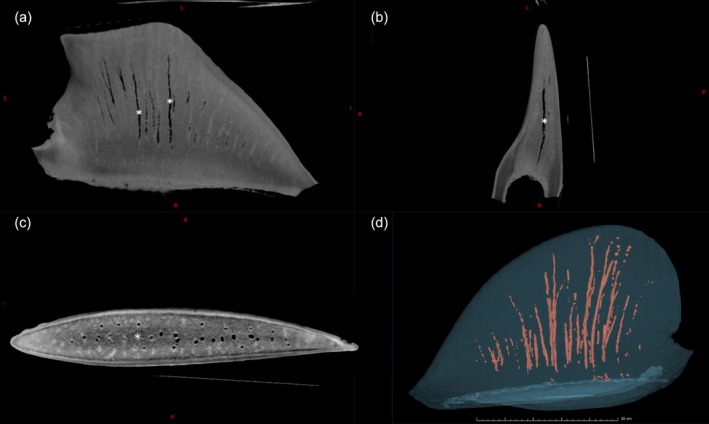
CT scan of the dorsal fin of spectacled porpoise (*P. dioptrica*) KS14‐45Pd/X2020.76. (a), (b) and (c) show the sagittal, transversal, and axial planes at its base, respectively. (d) The 3D reconstruction with the render of the arteries. In (a), (b) and (c), the asterisks highlight the central arteries. These are shown as hypodense (dark) regions. The circumferential veins surrounding the arteries, due to their weaker wall and size, may have collapsed, thus appearing as small hyperdense areas. Conversely, the peripheral veins were visible as hyperdense areas.
